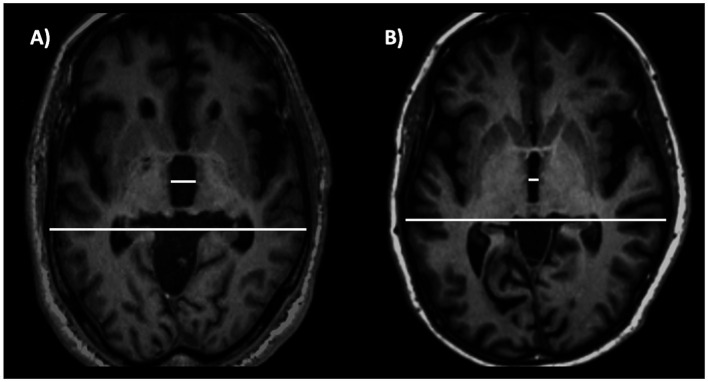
A 3T brain T1-weighted axial MR image at the level of the third ventricle’s maximum dilation, showing how to measure the third ventricle width. This measure is normalized by the internal skull diameter on the same axial slice. The figure shows this measure in a patient with progressive supranuclear palsy (A) and in a patient with Parkinson’s disease (B). This structure is enlarged in progressive supranuclear palsy compared to Parkinson’s disease.
